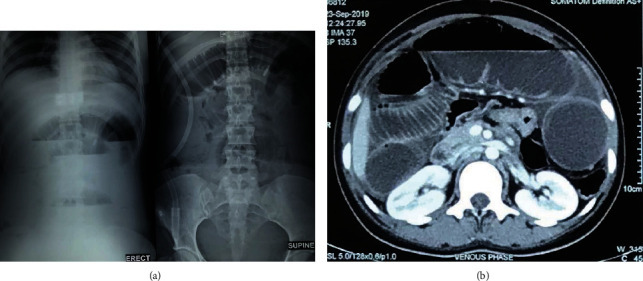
(a) X-ray showing air fluid levels in the left and dilated loops of small bowel with prominent valvulae conniventes suggestive of small bowel obstruction. (b) CECT scan showing dilated bowel loops suggestive of intestinal obstruction.
